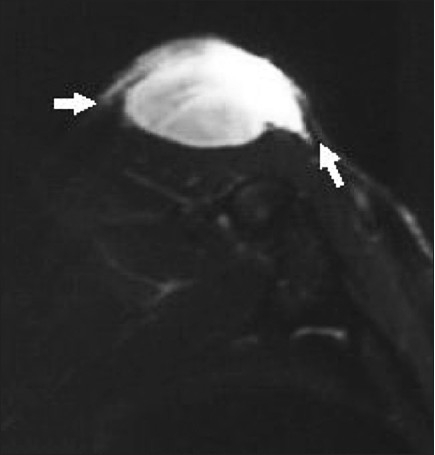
Tail-like projections in fibrosarcoma protuberans in fat- saturated T_2_-weighted axial MR image. The tumor with its high-signal-intensity pattern shows tail-like deep extensions (arrows) with some indefinite contours
